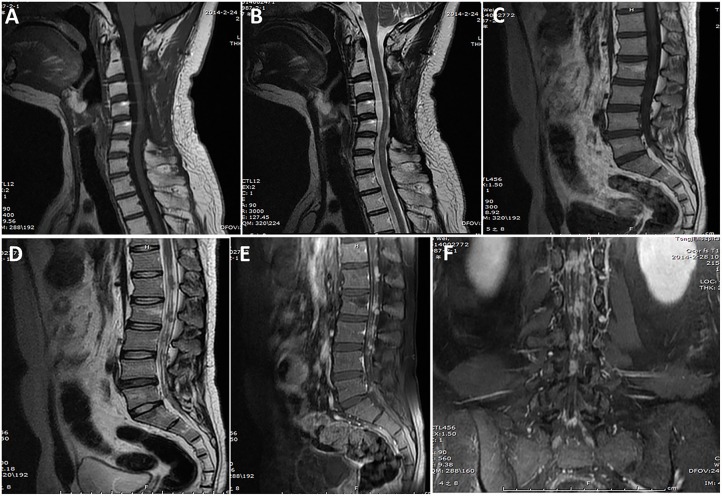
A case of primary intradural ES/pPNET at the C3–C5 level. Images obtained 14 months after the first surgery (A,B) showed no tumor local recurrence at the C3–5 level (lack of preoperative MRI examination findings), but they did show multiple metastases in the spinal canal through the cerebrospinal fluid (C–F).
